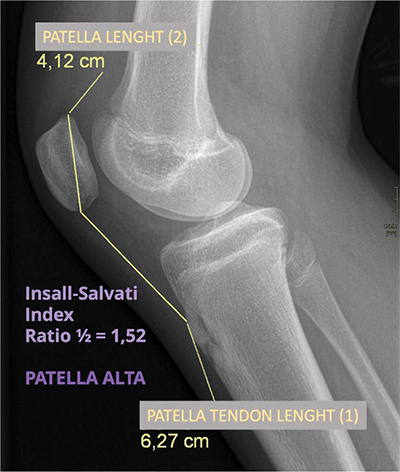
Lateral radiograph of the knee. Joint effusion and patella alta.
